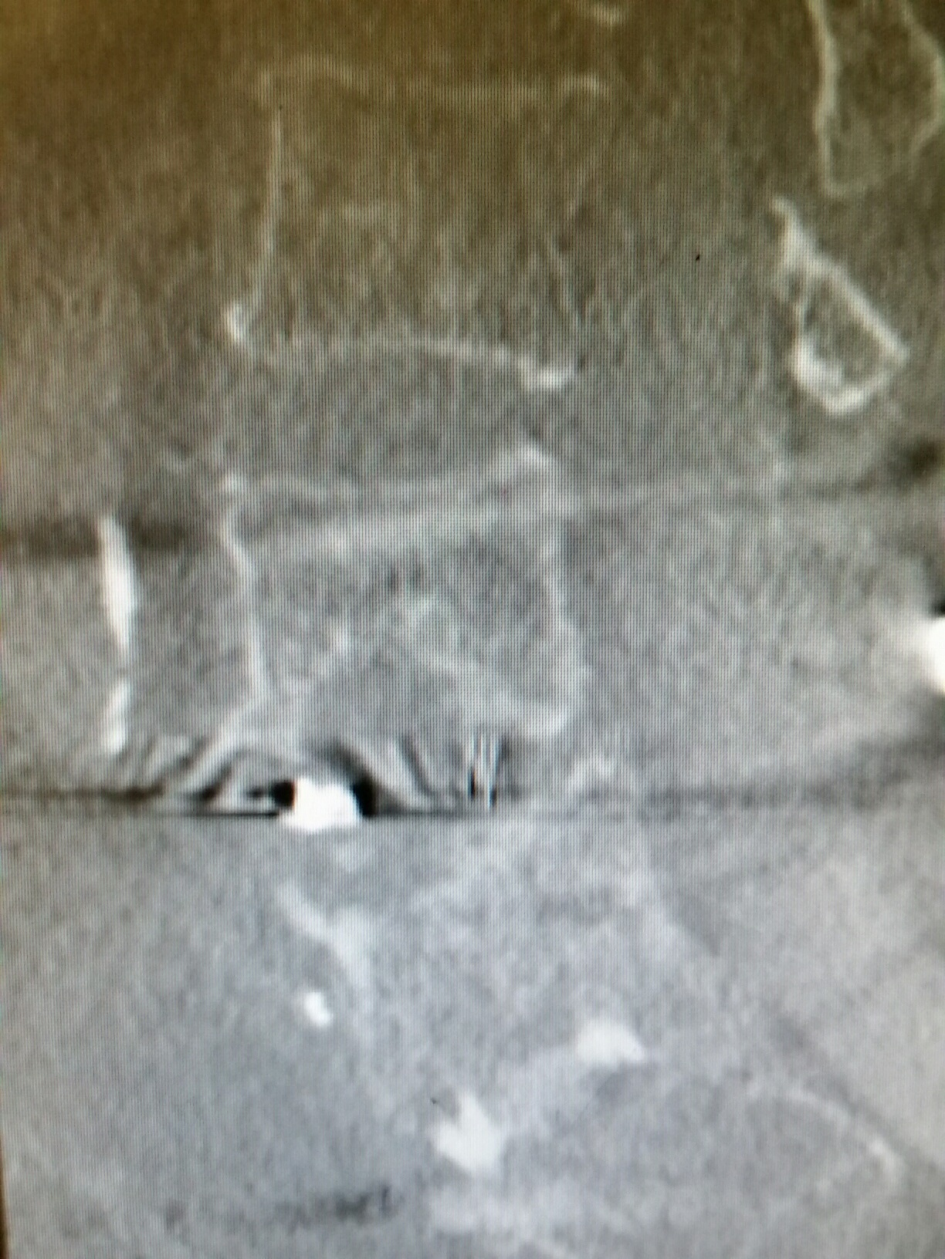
Sagittal CT reconstruction shows erosion of the endplates and rotation of the graft.
